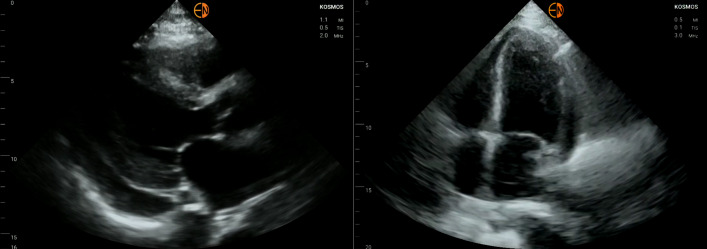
Parasternal long axis (left) and apical four chamber view (right). The left image shows a moderate sized pericardial effusion. In both images the walls were thickened and function was depressed towards the base of the heart. A subsequent echocardiogram described the findings as concerning for amyloid cardiomyopathy.
